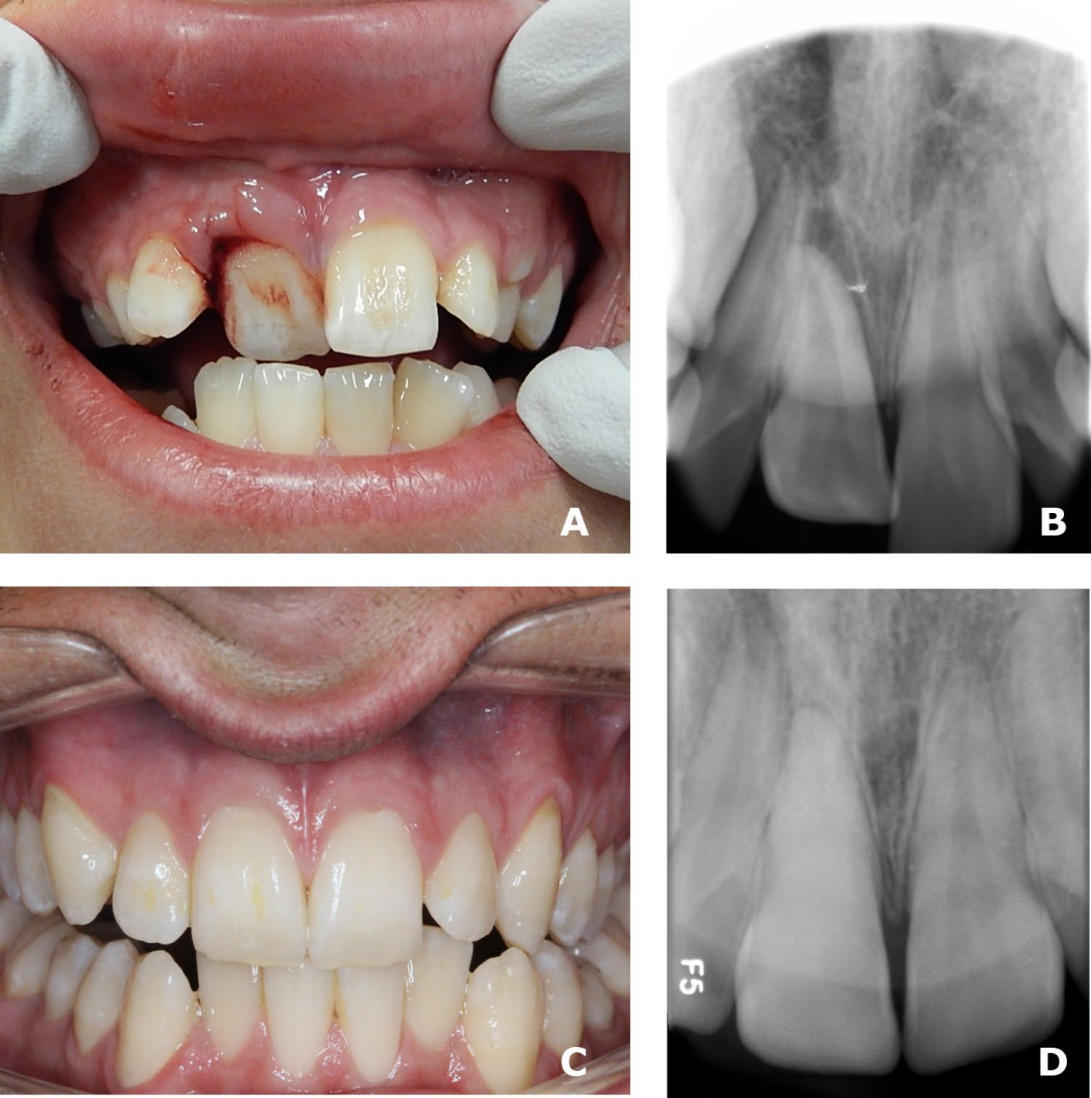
An example of a patient from the cohort from 2012 to 2020. The patient was diagnosed with a lateral luxation of 11. 3A A clinical photo, and 3B a radiograph before initial treatment was performed. 3C Clinical photo and 3D radiograph taken at the 1 year follow-up visit. PCO occurs in 11. Note that there is no yellow discoloration of the tooth crown. The tooth had a positive response to the pulp sensibility test. It was concluded that pulp healing occurred in 11
